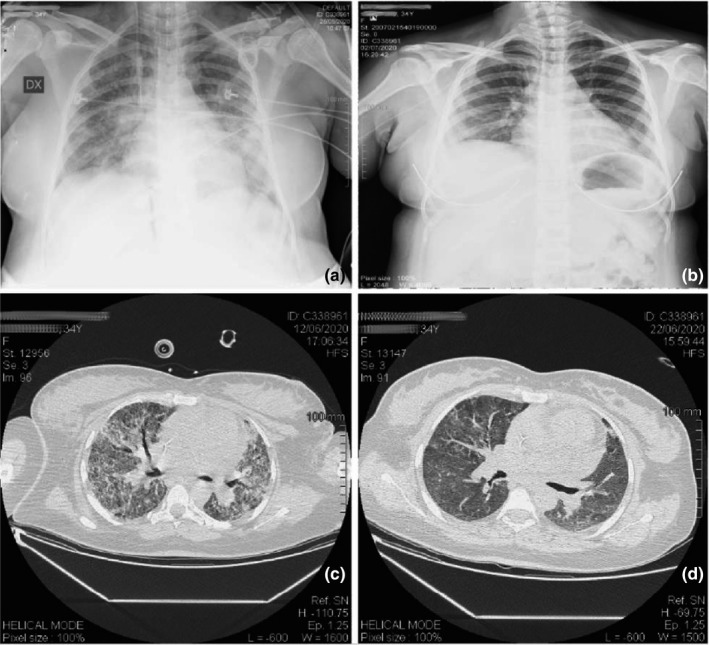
Chest radiographs and Computerized tomographies. The figure shows chest radiographs and chest computerized tomographies (CTs) at different moments. Panel A: Chest radiographs (day 3 from hospital admission) showing multiple bilateral parenchimal opacities tending to confluency, major on the left side. Panel B: Chest radiographs (day 38 from hospital admission) showing residual increase of bronchovascular markings in the basal region, more evident on the right side. Panel C: Chest CT (day 18 from hospital admission) showing multiple bilateral diffuse lung consolidations with ground glass aspect and thickening of lung interstitium. Panel D: Chest CT (day 28 from hospital admission) showing residual consolidations with fibrotic aspect in the upper lobes and diffuse thickening of lungs' interstitium with ground glass prevalent pattern
